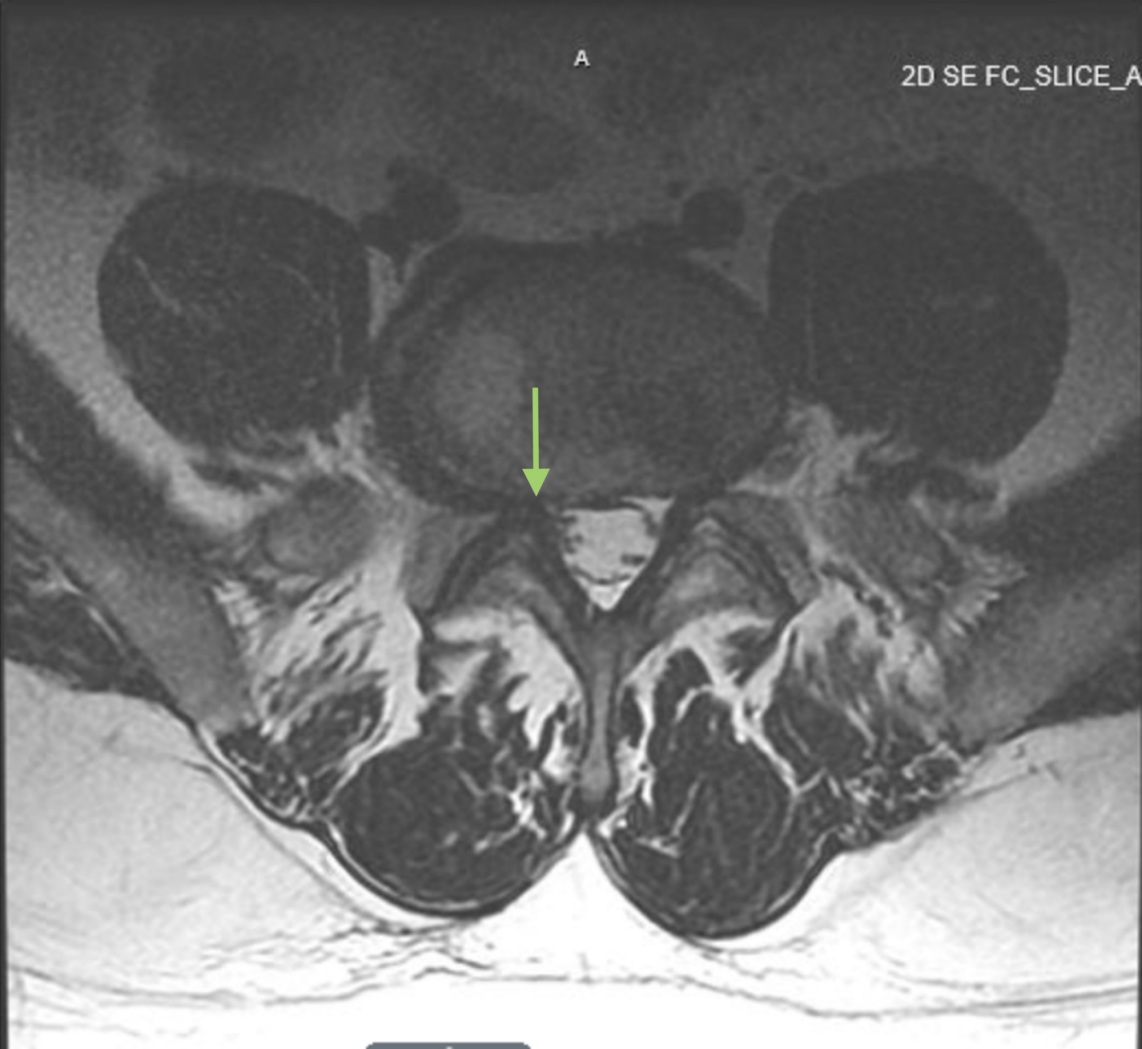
MRI lumbar spine T2-weighted axial image. Arrow showing L5-S1 disc herniation causing narrowing of the right neural foramen and impingement of the exiting L5 nerve root.
